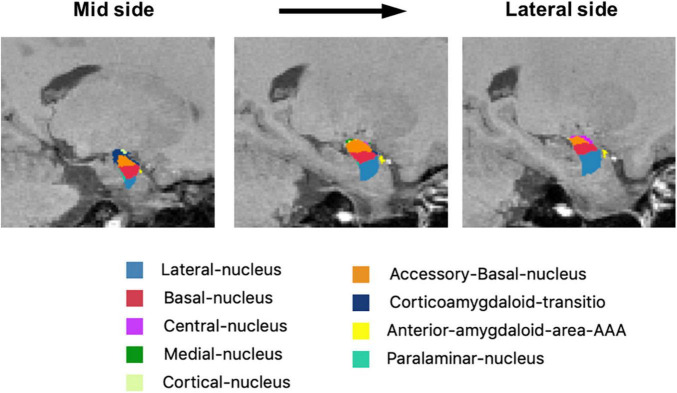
Representative images of the amygdala subregions. The mask of each region overlaid on sagittal T1-weighted images from mid side to lateral side.
